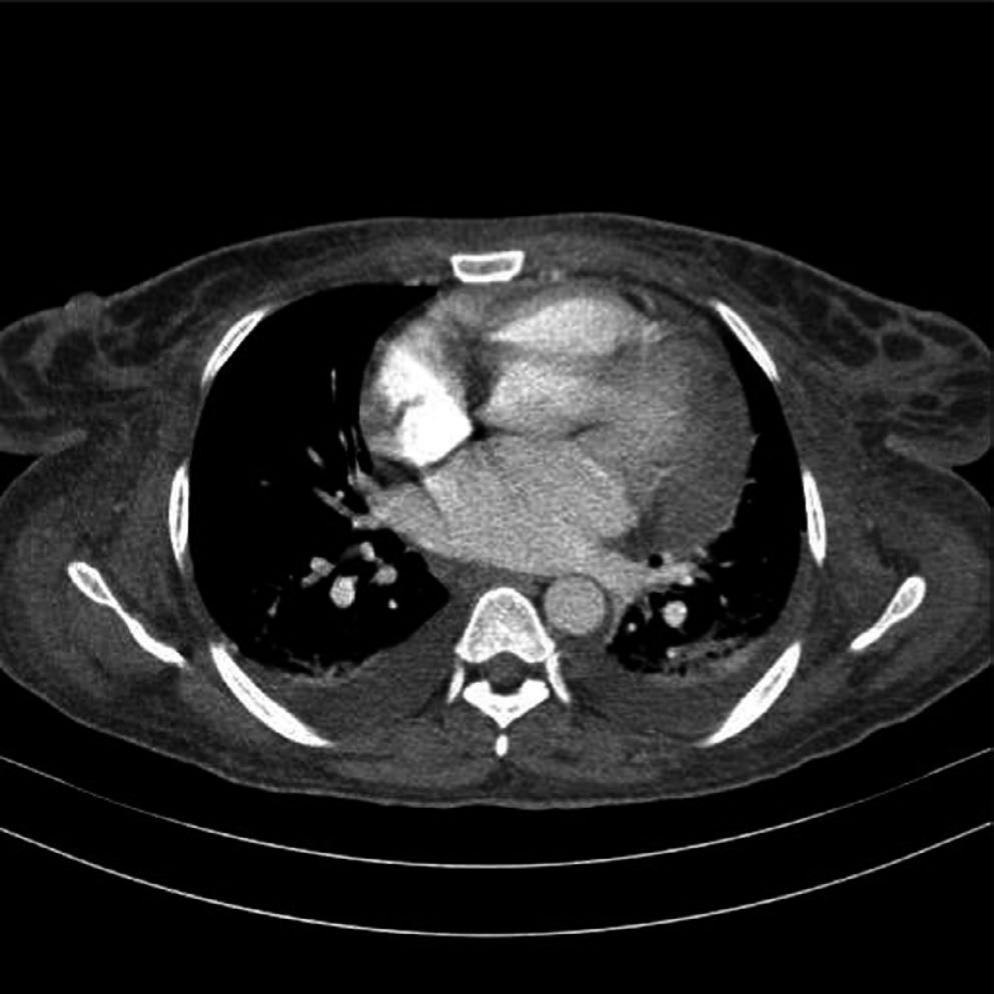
Axial thoracic CT scan cut of lower thoracic region set at thoracic window; in this cut pericardial effusion is also noticeable
